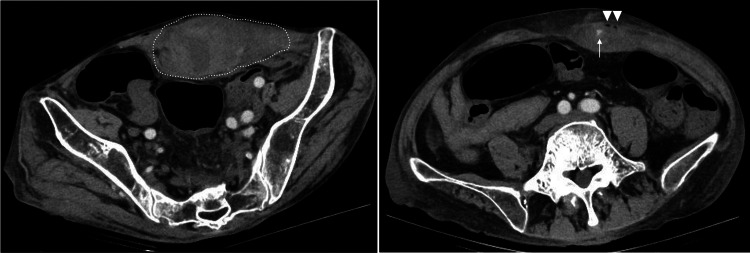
Contrast-enhanced computed tomography (a,b) There was a high-density left-sided RSH of 120 mm × 40 mm (a, dotted line), with small amounts of gas (b, arrow head) and extravasation of contrast media (b, arrow).
